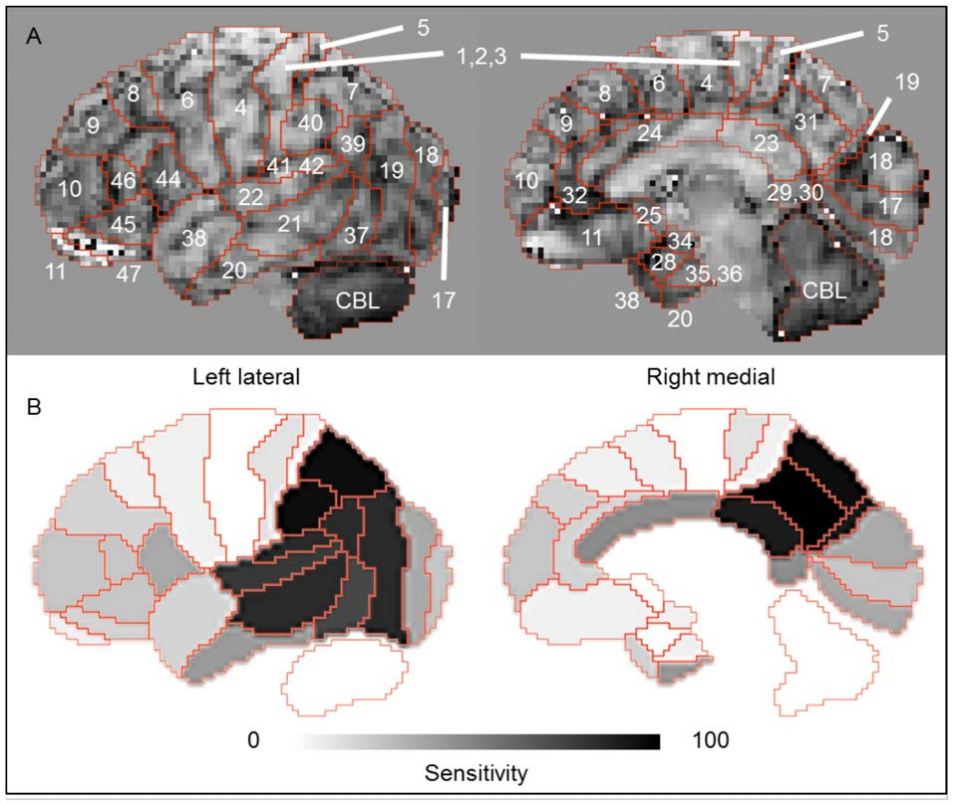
BA on 3D-SSP images. BA on 3D-SSP MRI template images (A) and sensitivity-distribution maps of BA on 3D-SSP images among NL and AD in Category A (B). The color bar denotes the levels of sensitivity to differentiate NL and AD in Category A.
